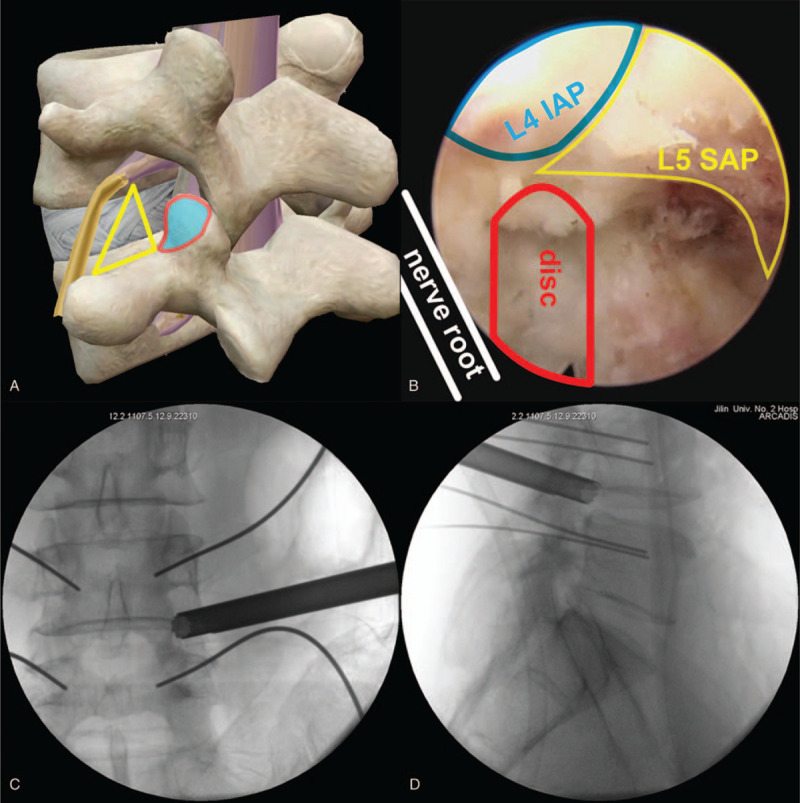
The yellow triangle represents Kambin's triangle and the bone in the red circle is part of the L5 that needs to be resected (A), the blue, yellow and red regions represent L4 inferior articular process and L5 superior articular process after partial resection (B), anteroposterior (C) and lateral (D) radiographs of the L5 superior articular process arthroplasty.
